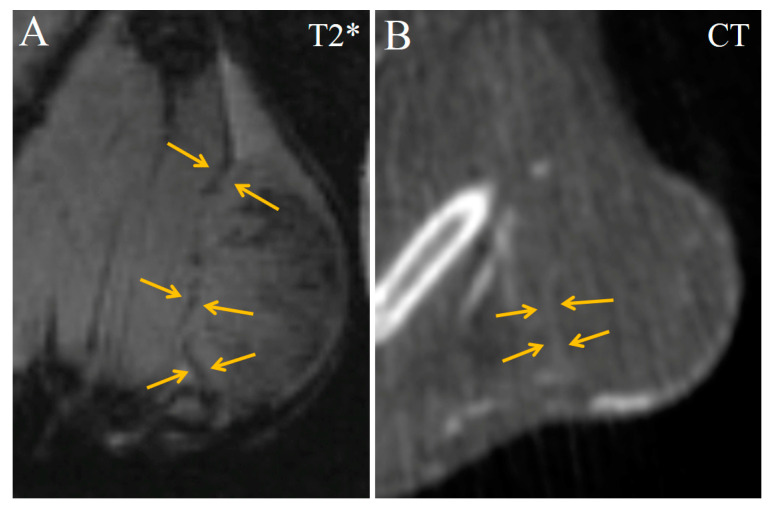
Coronal images of Ca755 mammary carcinoma. (A) T2*MRI 16 min post injection of Fe_3_O_4_@Au; (B) CT image 22 min post injection of Fe_3_O_4_@Au. Enhanced lines in the inner periphery (related to the tumor capsule) distinguish the tumor from adjacent muscle.
